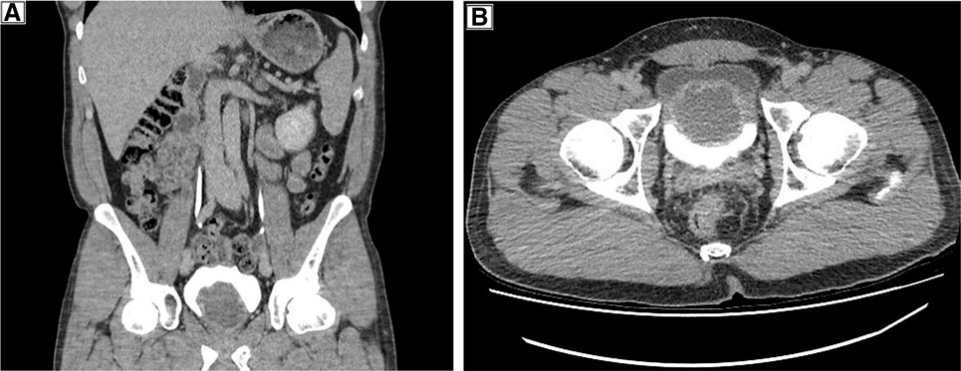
CT urogram showing the cystic bladder lesion. (A) Sagittal CT scan; (B) coronal cross-section showing the contrast-enhancing cystic bladder lesion.
